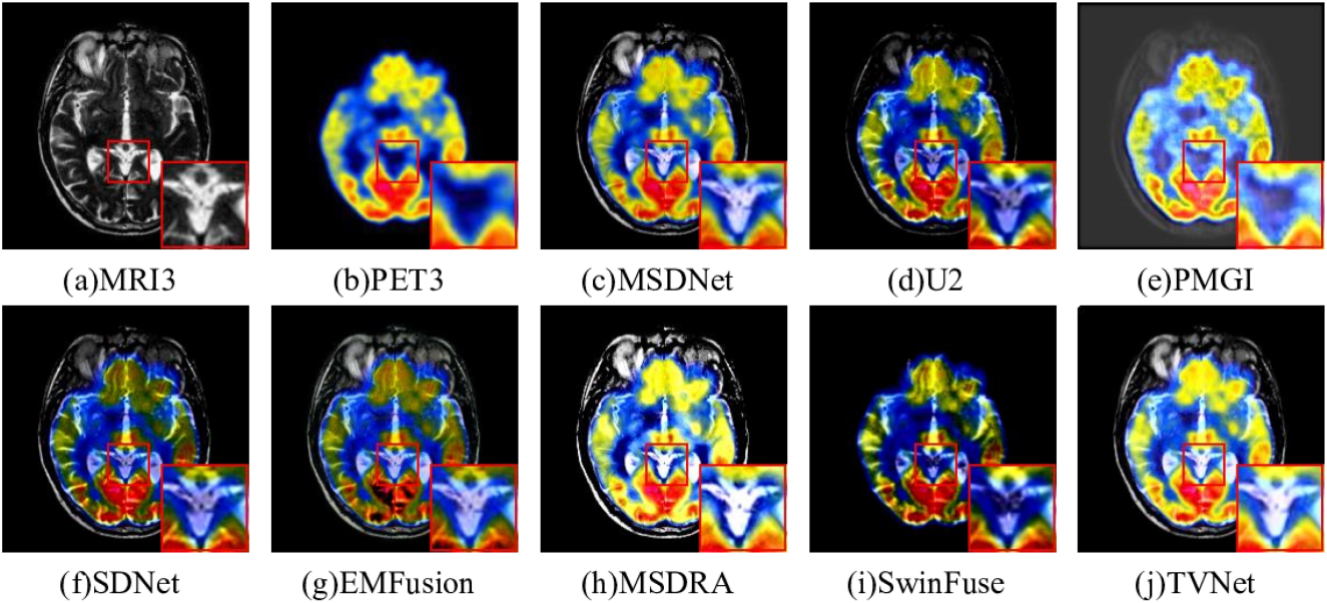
Comparison on magnetic resonance imaging (PET-MRI) fusion.
